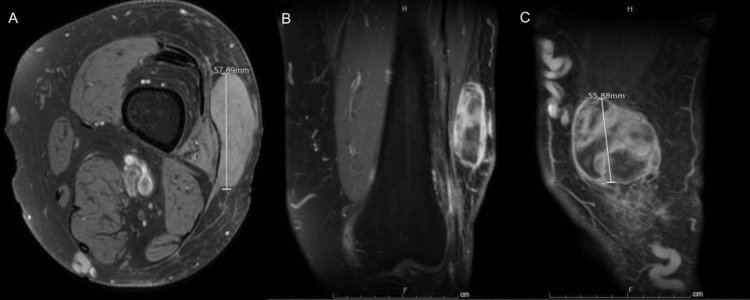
Magnetic resonance imaging (MRI) with and without contrast showed heterogeneous solid enhancing soft tissue mass A) - axial view B) - coronal view C) - sagittal view.
